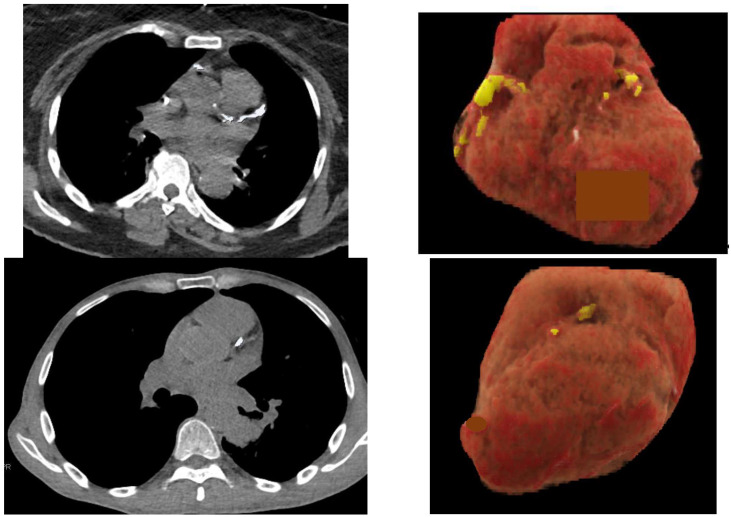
Ungated non contrast chest CT scans in two different smokers with automatic quantification of coronary artery calcifications (CAC) and heart volume using the AI-RAD Companion (Siemens Healthinners) software. Axial CT images show the CAC (left) and coronal 3D projection of the heart (right) that shows the segmented CAC (yellow). The total CAC and heart volumes were 1175 mm^3^ (category IV) and 620 mL, respectively, in the first subject (top), and 187 mm^3^ (category III) and 832 mL, respectively, in the second (bottom).
